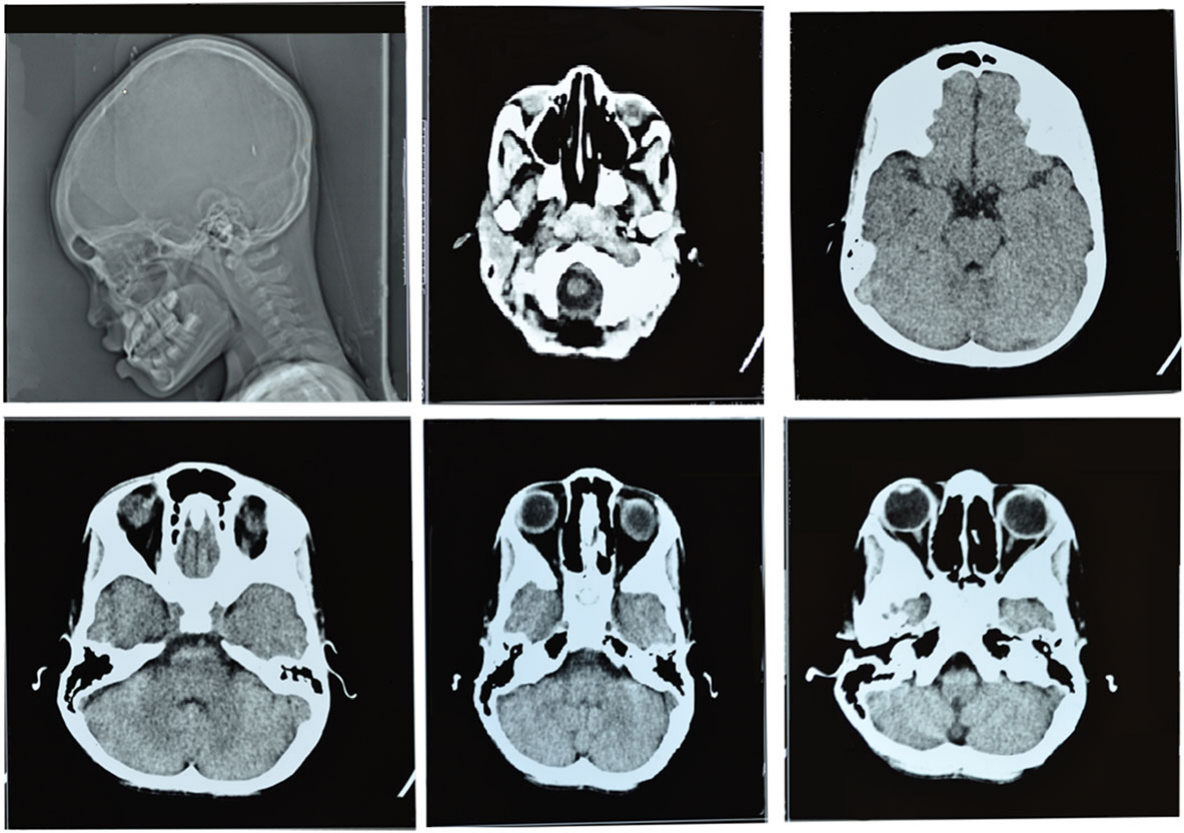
CT scan images of subperiosteal orbital bone abnormality
